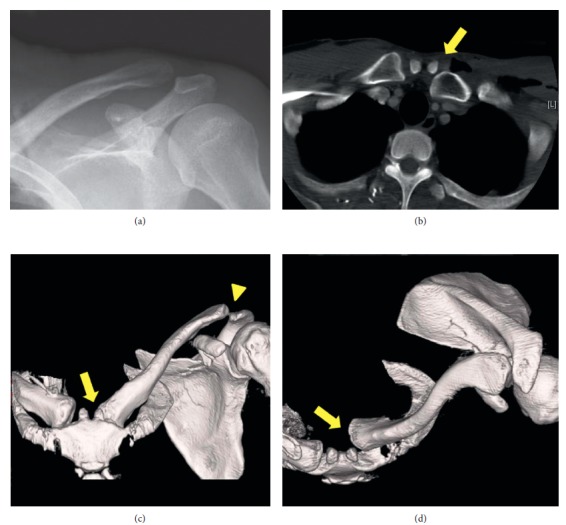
Case 2: images at the first presentation. (a) A radiograph shows type III ACJ dislocation. (b) A computed tomography (CT) scan shows posterior SCJ dislocation (arrow) as well as massive subcutaneous emphysema. (c) and (d) Three-dimensional reconstructed CT images of the left clavicle, which were made after the diagnosis was confirmed, clearly show ACJ dislocation (arrowhead) and posterior SCJ dislocation (arrow).
